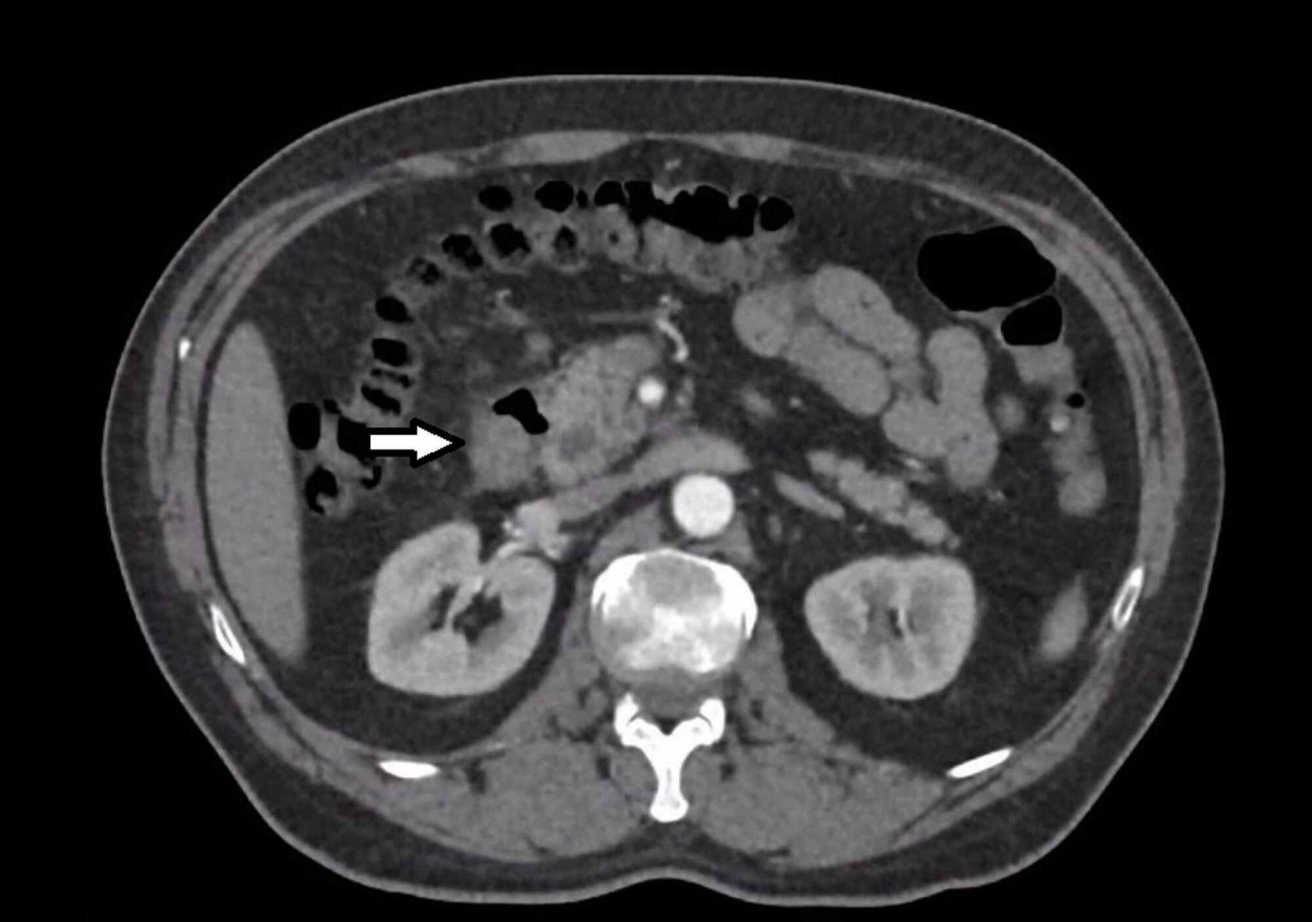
CT abdomen (axial): Bilateral vena cava merging into the preaortic trunk. Note in proximity an apparent cavitation, with air fluid level, at the medial aspect of the second part of duodenum.
